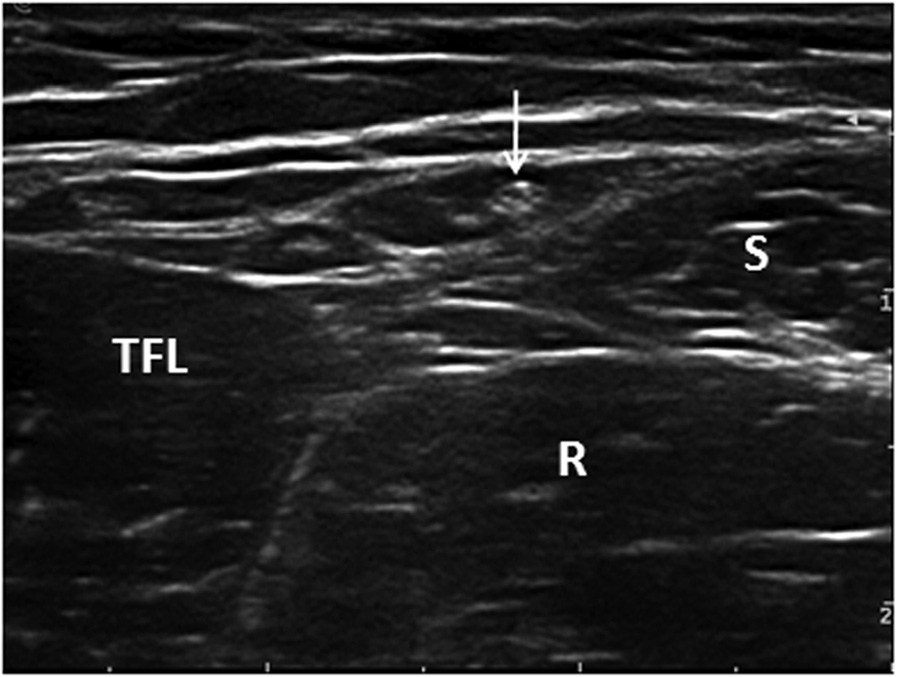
Transverse ultrasound image of the LFCN lying within the intermuscular space between the tensor fasciae latae muscle and the sartorius. LFCN: lateral femoral cutaneous nerve; TEL: tensor fasciae latae muscle; S: Sartorius; R: rectus femoris.
